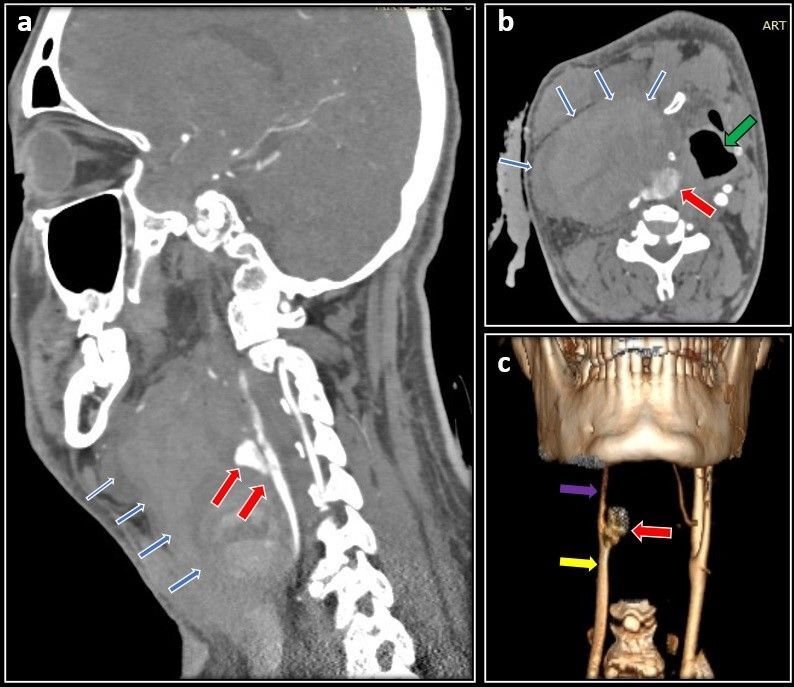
CT Angiography images after pseudoaneurysm formation. A) Sagittal cut showing a large hematoma (blue arrows) along with the pseudoaneurysm (red arrows) arising from the CCA; B) Axial cut showing the tumor blush (red arrow), and hematoma (blue arrows) causing severe compression of the trachea (green arrow); C) Reconstructed image shows the CCA (yellow arrow), attenuated ICA (violet arrow), and aneurysm (red arrow) at the carotid bifurcation with non-visualization of the ECA.
